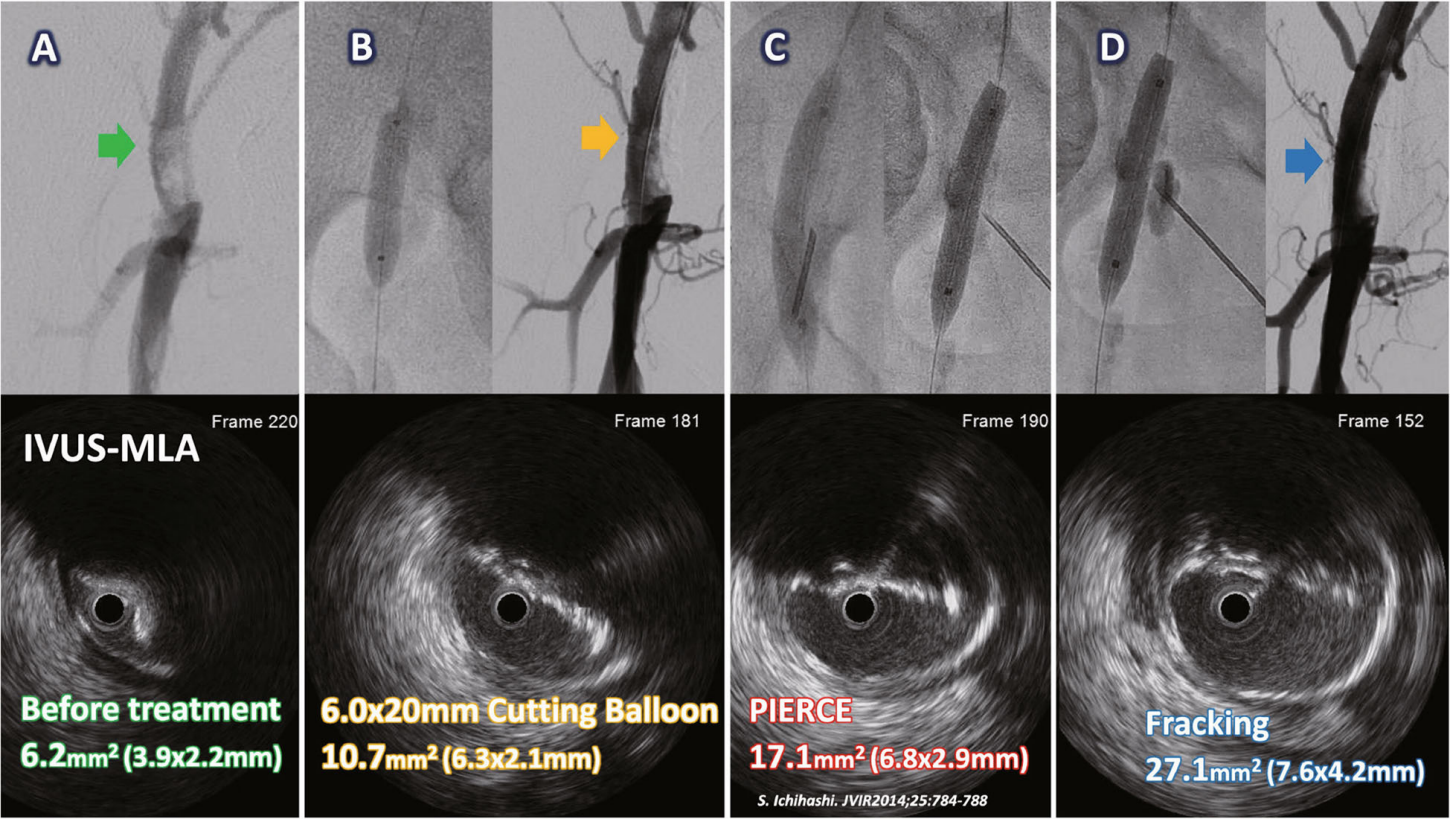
Angiography and intravascular ultrasound (IVUS) of the treatment with the fracking technique in case 1. A Control angiography for quantitative vessel analysis (QVA) showed stenosis of 94% in eccentric calcified plaque in the right common femoral artery (green arrow), and IVUS before treatment showed a minimal lumen area (MLA) of 6.2-mm^2^. B After dilatation with a 6.0 × 20-mm cutting balloon, QVA showed 24% stenosis (yellow arrow), and the MLA was 10.7-mm^2^. C After a 7.0 × 40-mm noncompliant balloon inflation and percutaneous direct needle puncture of calcified plaque (PIERCE) to modify the calcification (Ichihashi et al. 2014), the MLA was 17.1-mm^2^. D After the fracking was repeated at three locations at up to 8-atm, QVA showed an improvement of stenosis to 16% (blue arrow), and the MLA was significantly much greater, to 27.1-mm^2^
